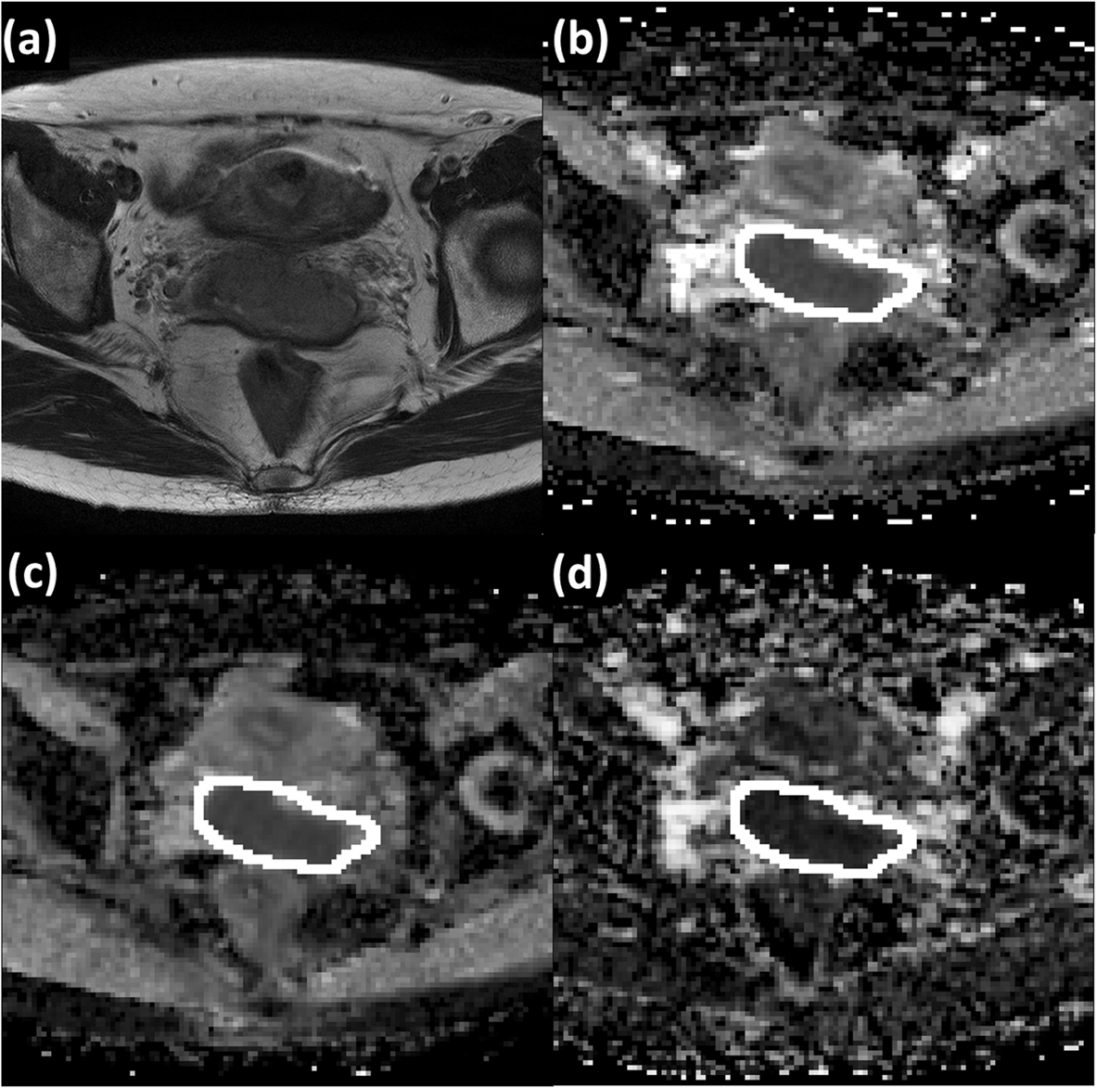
Representative example of the placement of region of interest in the parametric maps of (a) T2-weighted image, (b) apparent diffusion coefficient (ADC), (c) pure diffusion coefficient (D), and (d) perfusion fraction (*f*) on a case of a 66-year old patient International Federation of Gynaecology and Obstetrics (FIGO) staged IIA2. This was repeated on subsequent slices to include the entire tumour volume.
